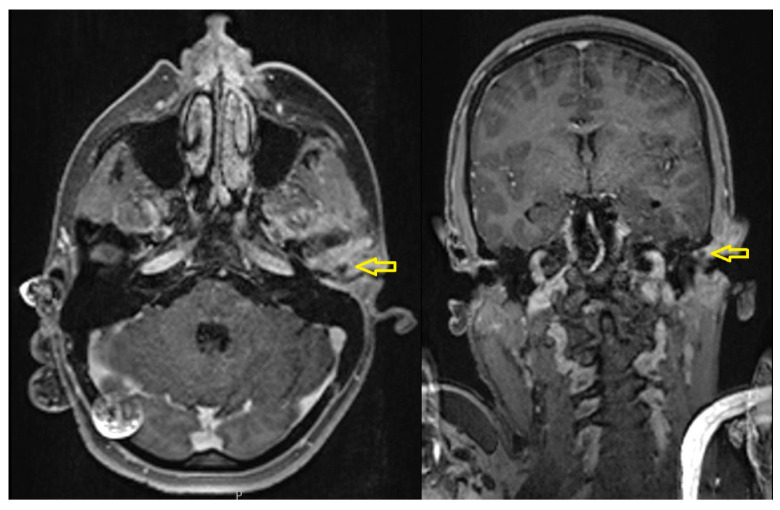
Axial (left) and coronal (right) contrast-enhanced MRI images showing effusion in the left temporomandibular joint (TMJ) and surrounding phlegmonous inflammatory changes (yellow arrows).
